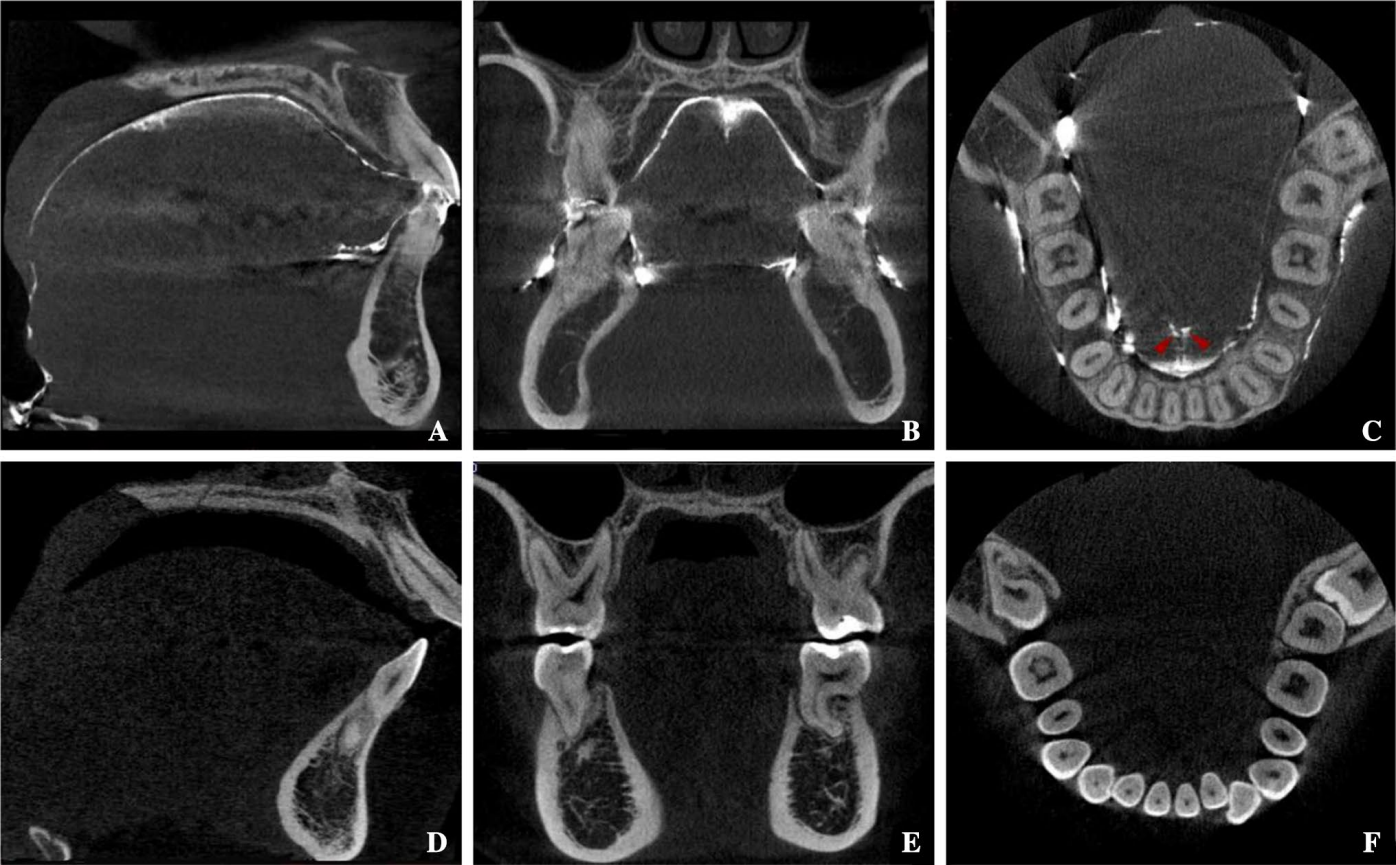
CBCT images of the oral cavity. a–c CBCT images of the oral cavity coated with radiocontrast agent. A clear border line is visible. Red arrows indicate the lingual frenulum. d–f CBCT images of the oral cavity without radiocontrast coating. The boundary of the tongue is indistinct, and the lingual frenulum is not distinguishable
